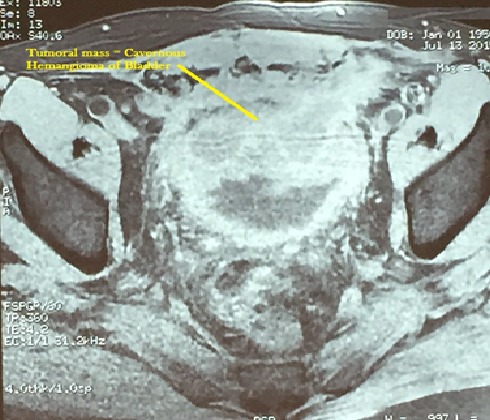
Transverse section in MRI of the CHB: T1 gadolinium injection
